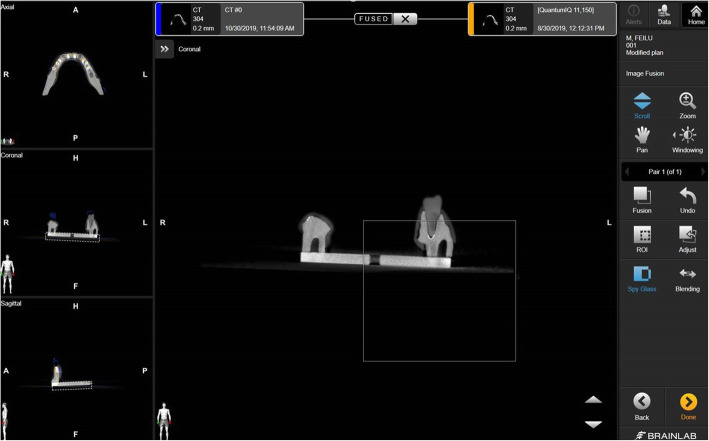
CBCT images of test model and master model were fused based on the metal base
